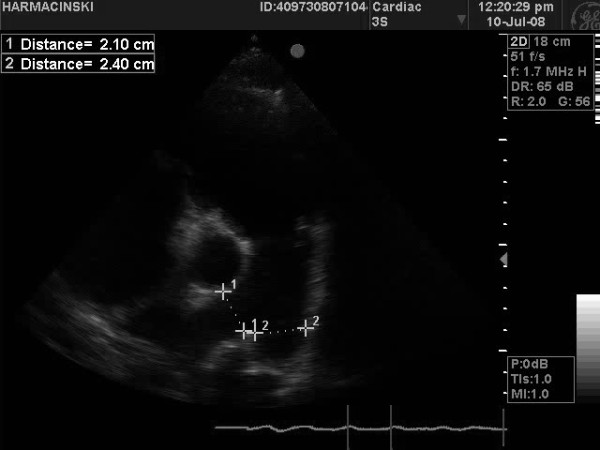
The demonstration of pulmonary artery trunk diameter measurement (tranthoracic echocardiography).
